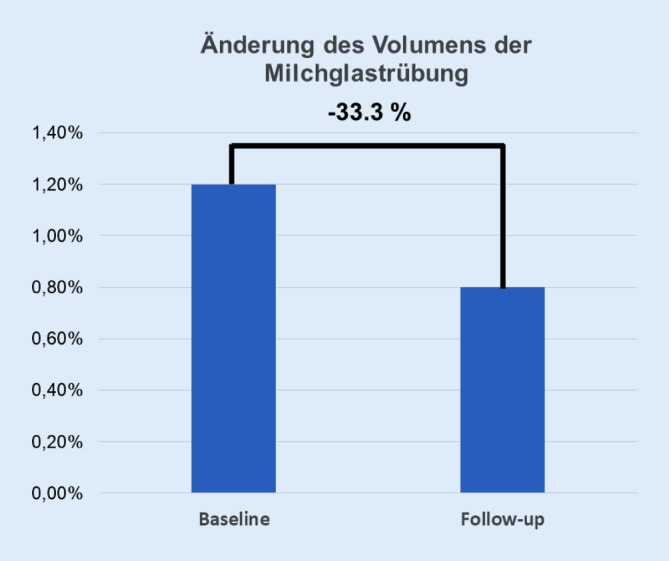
Darstellung der Abnahme der Milchglastrübungen mittels auf künstlicher Intelligenz basierender Quantifizierung der pulmonalen hochauflösenden Computertomographie („artificial intelligence-based quantification of pulmonary HRCT“ [AIqpHRCT]) im Verlauf über 6 Monate unter der Therapie mit Tofacitinib
